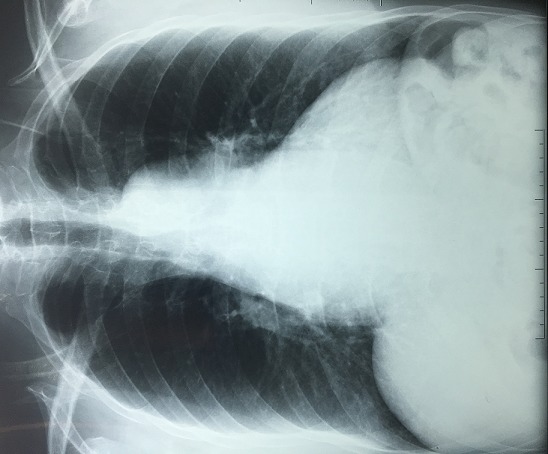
Radiographie pulmonaire de face montre un élargissement médiastinale
